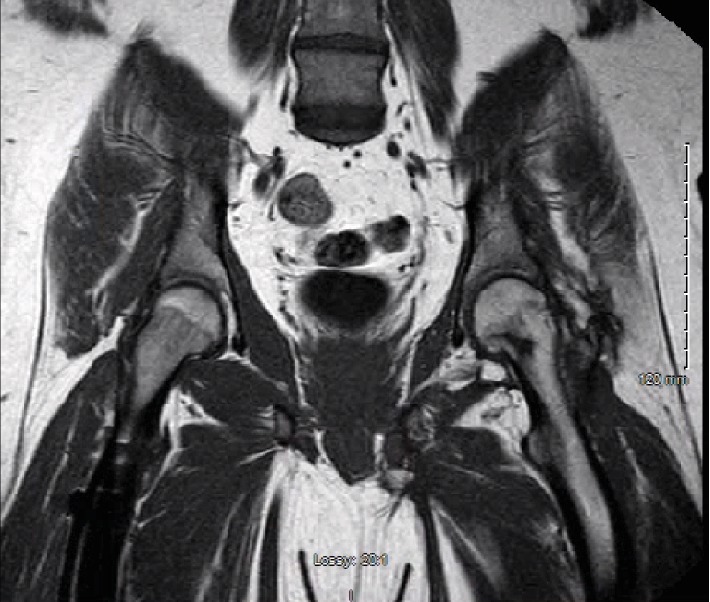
T1 coronal image from MRI obtained 7 months postinjury demonstrating focal areas of hypointensity within the left inferior femoral neck consistent with osteonecrosis.
